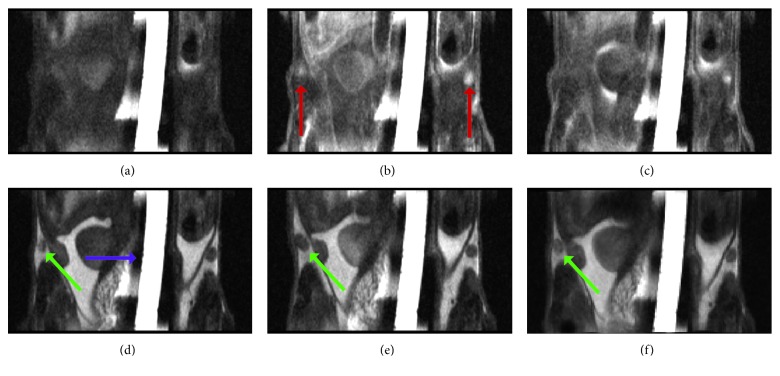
Precontrast (a), early (b), and late (c) enhancement of DCE-MRI images acquired, respectively, before 80 and 800 seconds after the administration of Gd-BOPTA and after a motion correction procedure. The lymph nodes are indicated by red arrows. Unsaturated fat T1-weighted images (same slice as (a)–(c)) before (d) and about 50 minutes after (e) the administration of the contrast agent, i.e., after the acquisition of the DCE-MRI scan; (f) is the same as (e) after a nonlinear motion correction procedure. Green arrows indicate a large displacement of the left node from (d) to (e) that was not fully recovered in (f) and consequently was excluded from the successive analysis. Frames (d)–(f) also show that a fatty hilum is not present. The white strip indicated by the violet arrow is a vial filled by gadolinium solution used as a standard signal in all DCE-MRI sequences.
